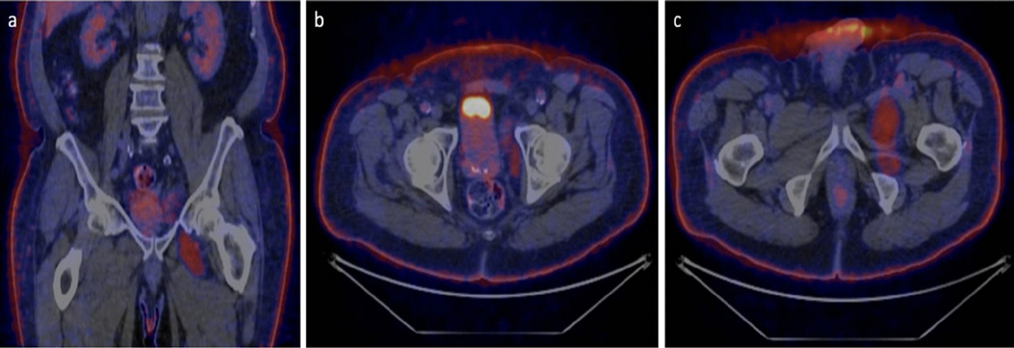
(a – c) PETCT (Positron emission tomography FDG with CT) demonstrating increased uptake within the entire lesion when compared with the background normal fat (no uptake). SUV of the lesion including the enhancing nodule/septation measured 6.0–7.8. (Lesions within SUVs of 2.0 are considered benign).
